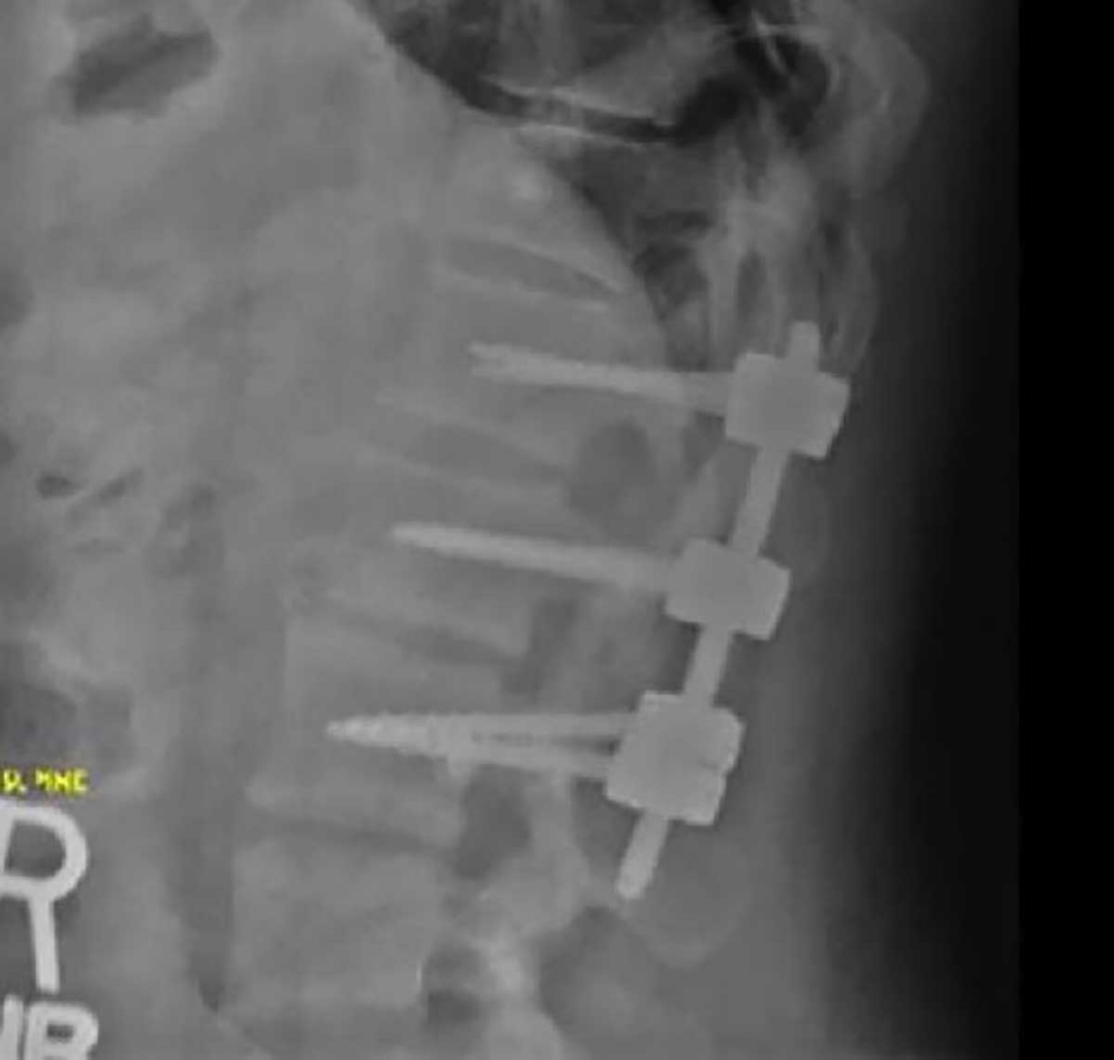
Postoperative lateral X-ray Good hardware placement and satisfactory reduction of the previously seen L1-2 distraction injury is seen.
